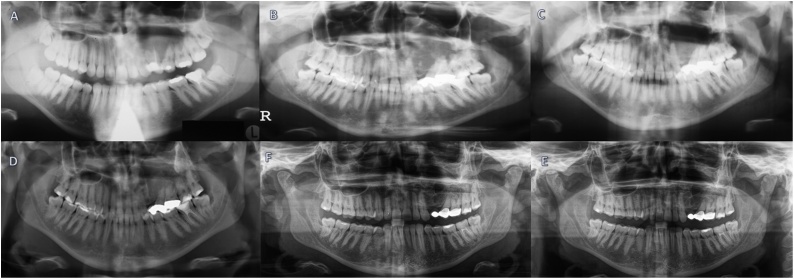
A,* orthopantomogram revealing a cystic lesion between teeth 23-26. *B,* preoperative orthopantomogram in year 2007. *E,* Postoperative orthopantomogram in year 2007. *C*, Postoperative orthopantomogram showing adequate removal of the lesion in 2010. *D*, orthopantomogram obtained in 2017 showing no signs of recurrence and good bone remodelling. *E*, orthopantomogram obtained in 2019. It is clear to see that the situation hasn’t changed during the years 2017 and 2019.
